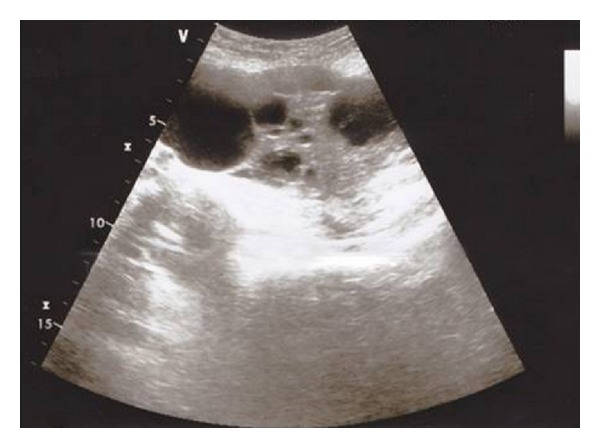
Endovaginal pelvic ultrasound: axial view: Adnexal mass measuring 20 cm, which is heterogeneous, with tissular and cystic components and without intracystic or extracystic exophytic vegetations and without peritoneal fluid.
